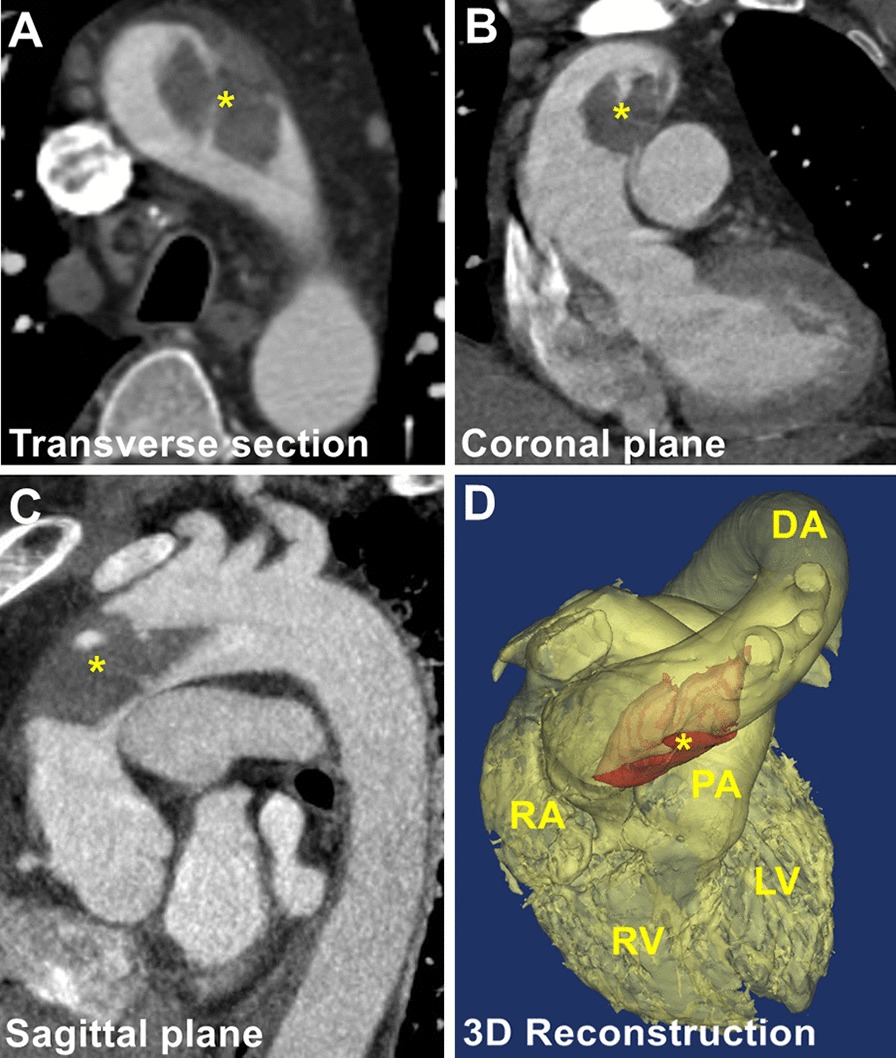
Preoperative CTA. Preoperative CTA shows a filling defect in the ascending aorta (yellow start). a transverse section; b coronal plane; c sagittal plane; d 3-D reconstruction. *LV* left ventricle, *RV* right ventricle, *RA* right atrium, *DA* descending aorta, *PA* pulmonary artery
